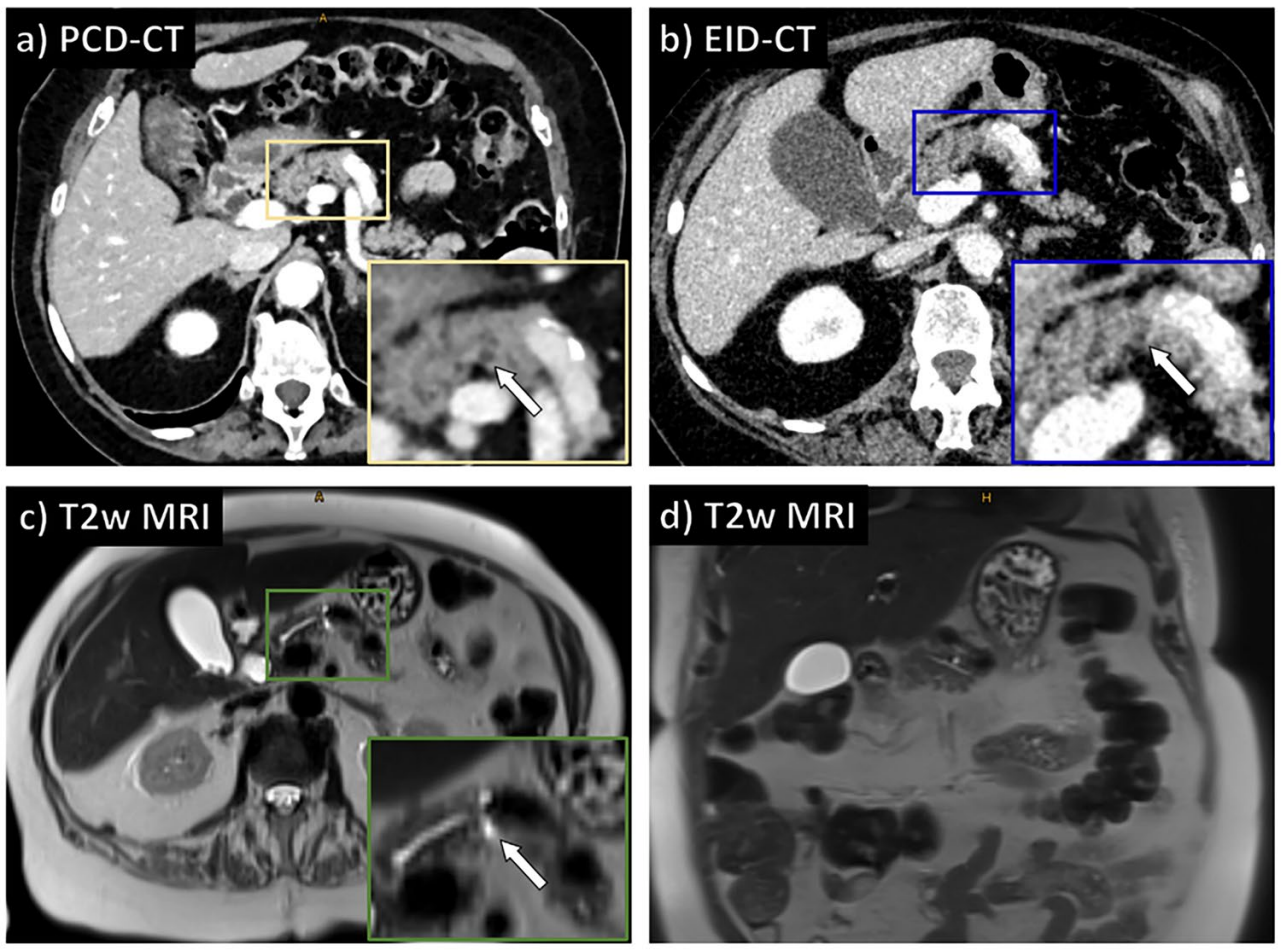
Image quality of PCD-CT vs. EID-CT for the detection of PCL. Images of an 83-year-old female patient who underwent repetitive MRI and CT imaging of the abdomen in the context of an osseous metastasized breast carcinoma. Axial PCD-CT reconstructions (a) show a small PCL (3 × 4 mm; white arrow) that is difficult to delineate in the EID-CT scan (b, white arrow). MRI references images clearly depict the PCL in axial (c) and coronal (d) T2-weighted images (white arrow). PCD-CT = photon-counting detector computed tomography; EID-CT = energy-integrating detector computed tomography
